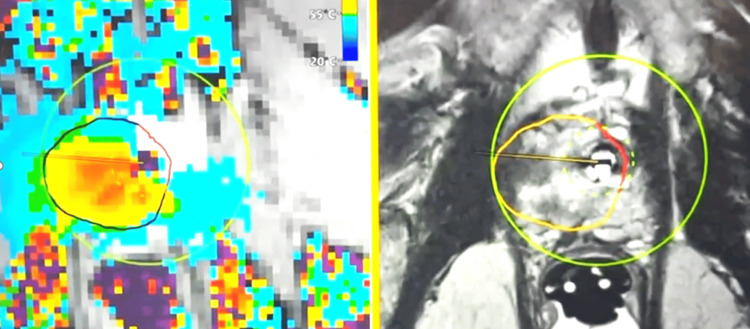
Initial treatment planning and "First-pass" ablation of an apical prostatic tumor T2-weighted image demonstrating a right apical prostatic tumor during real-time transurethral ultrasound ablation (TULSA) treatment. Colorimetric MR thermometry demonstrates ablative temperatures of 55°C-86°C within the planned treatment margin (orange line).
